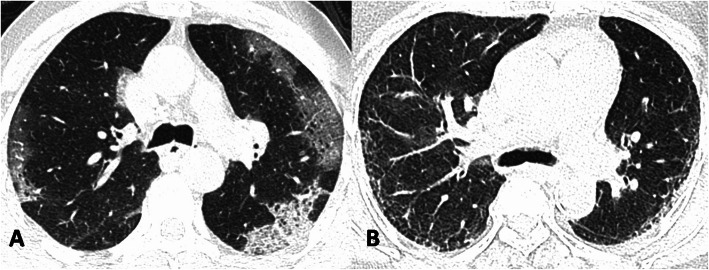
Axial chest CT image in lung window settings of a 51-year-old COVID-19 patient at the level of carina obtained at admission reveals elongated GGOs in the subpleural lung (a). Follow-up CT performed 107 days later reveals residual faint GGOs with interlobular septal thickening in the subpleural lung (b)
